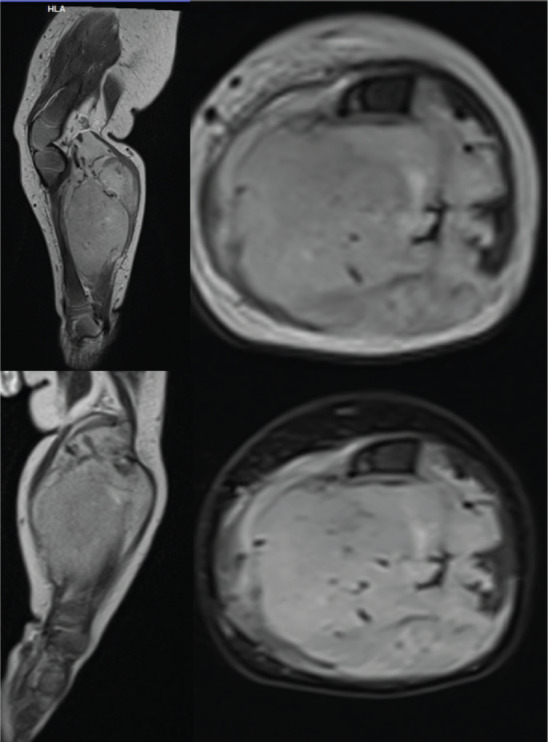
Pre-operative MRI images of the left leg showing a large lobulated invasive soft tissue mass lesion of 3.5 × 5 × 6 cm size in the upper and mid-leg involving postero-medial compartment with anterior displacement of the tibia with its bowing without any abnormal marrow signal change. Mass is hypointense on T1WI and heterogeneously hyperintense on T2WI. Mass is mainly involving the muscular structures with evidence of neuro-vascular encasement.
